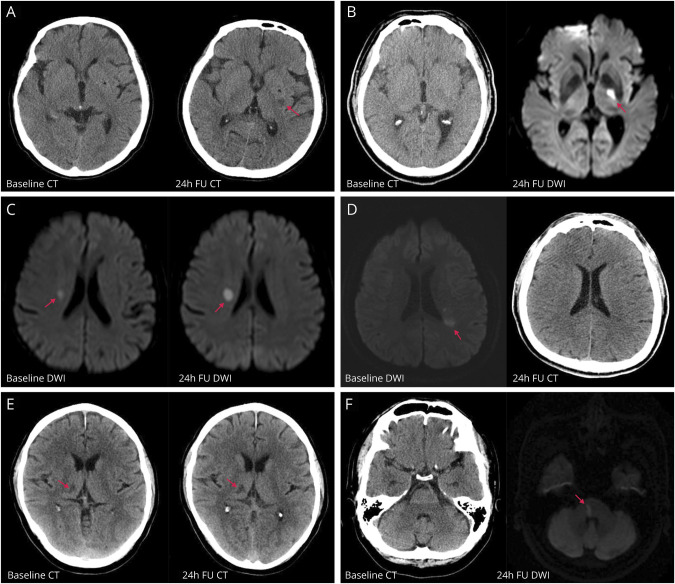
Examples of Lacunar Ischemic Stroke at Different Locations From ENCHANTED Lacunar stroke at (A) left lentiform (red arrow) identified on 24-hour follow-up CT; (B) left internal capsule (red arrow) identified on 24-hour follow-up MRI; (C) right centrum semiovale (red arrow) identified on baseline and 24-hour follow-up MRI; (D) left internal border zone (red arrow) identified on baseline MRI; (E) right thalamus (red arrow) identified on baseline and 24-hour follow-up CT; and (F) brainstem (red arrow) identified on 24-hour follow-up MRI. DWI = diffusion-weighted imaging; ENCHANTED = Enhanced Control of Hypertension and Thrombolysis Stroke Study.
